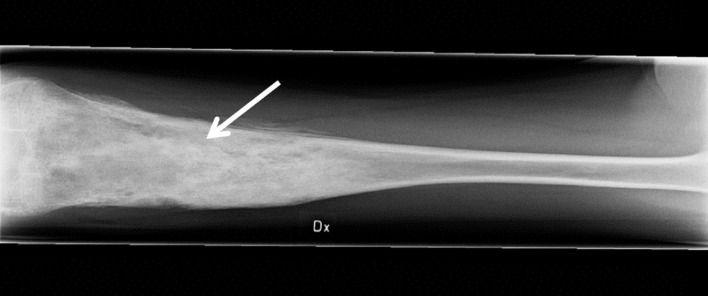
Inflammation of the femur. The anteroposterior radiograph is indicative of osteomyelitis (arrow) in the right (Dx) femur of the patient at age 14 years, prior to adalimumab treatment.
